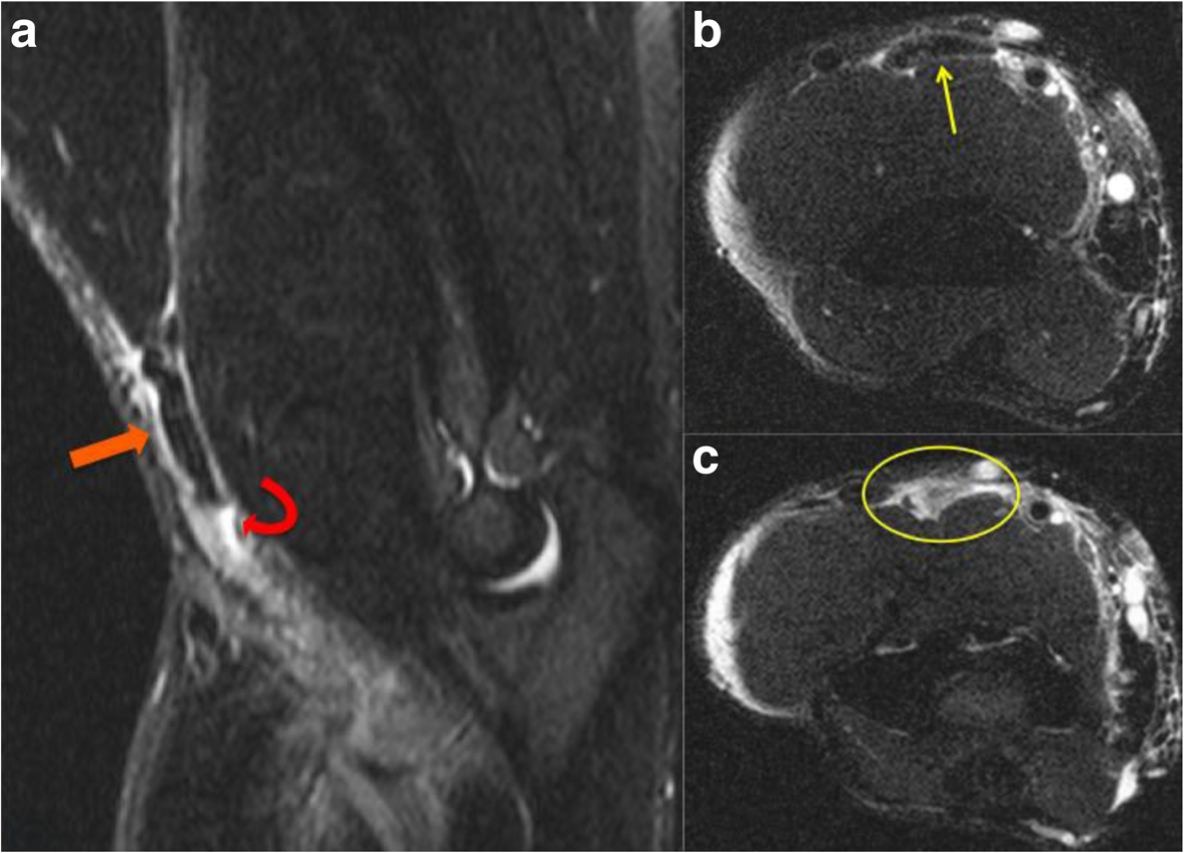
Magnetic resonance imaging of the right elbow. (a) Sagittal short tau inversion recovery image demonstrates a full thickness, complete tear of the distal biceps brachii tendon with proximal retraction (curved red arrow demarcates the retracted end of the torn tendon). Note the high signal intensity edema surrounding the thick, retracted tendon (thick orange arrow). (b,c) Axial fat-suppressed fast spin echo T2-weighted images demonstrate the thickened tendon superiorly (thin yellow arrow in b) with high signal fluid filling the defect left by the torn, retracted biceps brachii tendon more inferiorly (yellow oval in c).
